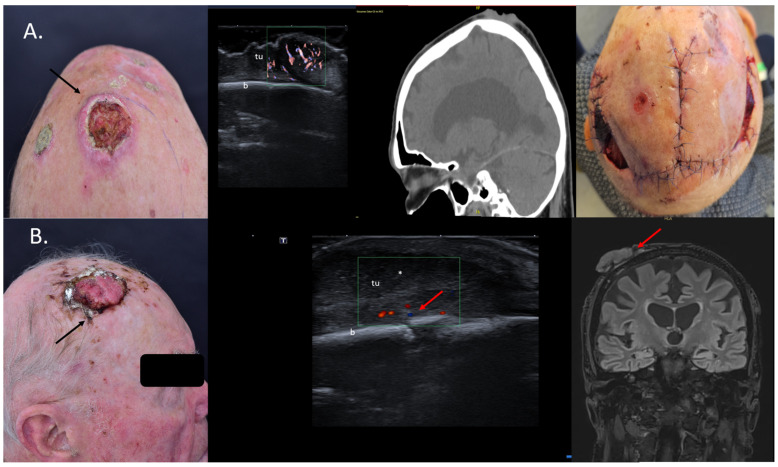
(Clinical aspect—left; HFUS, CT, MRI—middle; postoperative aspect—right): (A) Clinical aspect of an exulcerated tumor at the parietal area, as marked by “↑”, consistent with an SCC; HFUS and microvascular imaging showing an irregular hypoechoic, highly vascularized lesion (“tu”) extending up to the bony surface (“b”) with no bone infiltration—transverse view; CT scan showing no bone infiltration by the tumor mass; Clinical aspect after resection and partial defect closure with two rotation flaps; (B) Clinical aspect of an exulcerated SCC of the right parietal area, as marked by “↑”; HFUS and color Doppler display a hypoechoic lesion with suspicion of bone infiltration, as marked by “↑”—transverse view; MRI showing local bone infiltration, as marked by “↑”—coronal view.
